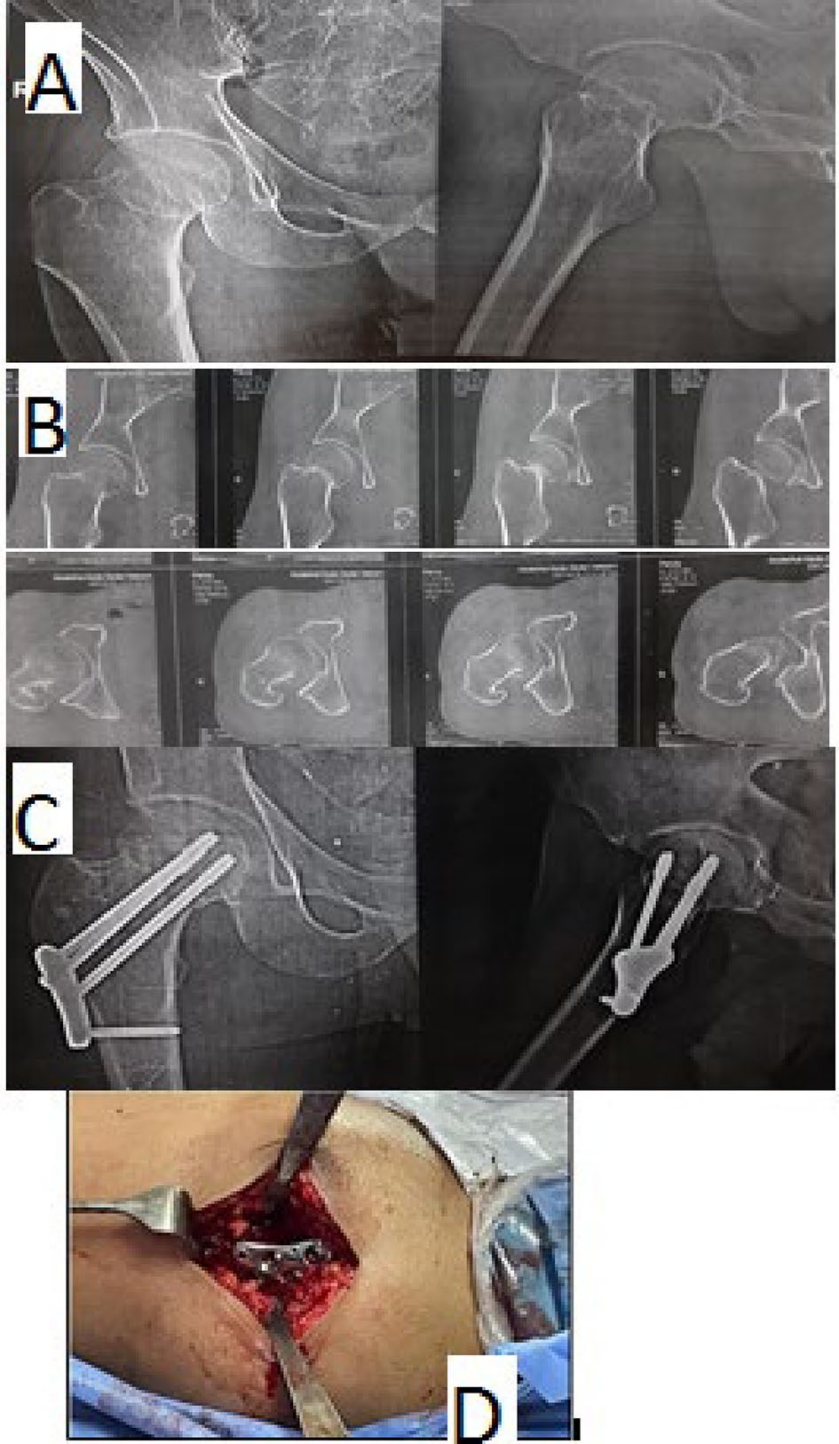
A 52 years old male patient with femoral neck fracture was resulting from road traffic accident and treated by FNLP: A Pre-operative plain x-rays showing subcapital femoral neck fracture of Garden type III and Pauwels type II. B CT scan. C immediate post-operative radiographs showing satisfactory reduction and fixation. D intra-operative clinical photo showing the surgical approach(B)Technique of multiple cannulated cancellous screws (MCCS):The initial inferocentral guide wire was positioned just above the lesser trochanter level, visible in both views. A parallel guide was then used to accurately place the posterosuperior and anterosuperior guide wires. Screw length was determined by measuring the guide wire and subtracting 5 mm. Predrilling of the holes for cannulated screws by appropriate cannulated drill pit. Three 6.5 mm partially threaded cancellous cannulated screws were utilized. Washers were also used as space allowed. (Fig. 2)Fig. 2 Fig2:
